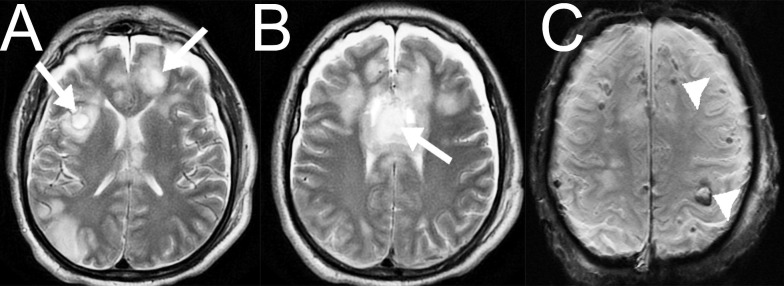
Case 3. Non-contrast magnetic resonance imaging (MRI) of the head. (A, B) Axial T2 weighted imaging (T2WI) and (C) susceptibility-weighted magnetic resonance imaging (SWI) axial slices showing multiple hemorrhagic contusions (arrows) in both frontal lobes as well as in the genu and anterior body of the corpus callosum with corresponding perilesional edema, as well as multiple cortico-subcortical hemorrhagic contusions (arrowheads).
